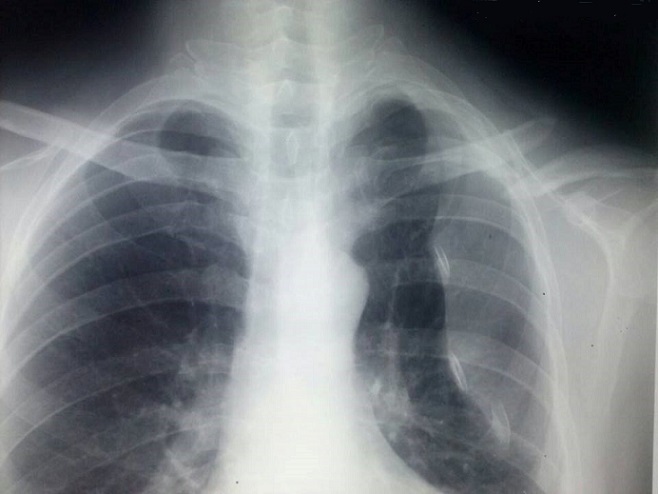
Chest radiograph taken two hours after the injury, showing left fractured clavicle and pneumothorax
